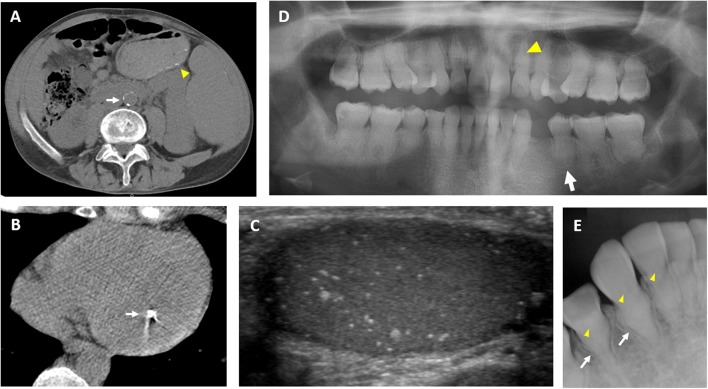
Clinical images of extraskeletal features in hyperphosphatemic tumoral calcinosis. (A) Computed tomography scan from a 36-year-old man demonstrates calcification in the abdominal aorta (white arrow). Small submucosal bowel calcifications are also visible (yellow arrowhead). (B) Computed tomography scan of the heart in a 29-year-old man demonstrates a calcified lesion in the papillary muscle (arrow). (C) Testicular ultrasound from a 32-year-old man showing diffuse microlithiasis. (D) Panoramic dental radiograph from an 18-year-old woman shows teeth with short, bulbous roots (white arrow) and obliteration of dental pulp (yellow arrowhead). (E) Periapical dental radiograph from a 12-year-old girl shows thistle-shaped root (white arrows) and obliteration of dental pulp (yellow arrowheads). These figures are original, and have not been previously published or obtained from a private database. There is no unpublished data in any of the figures.
